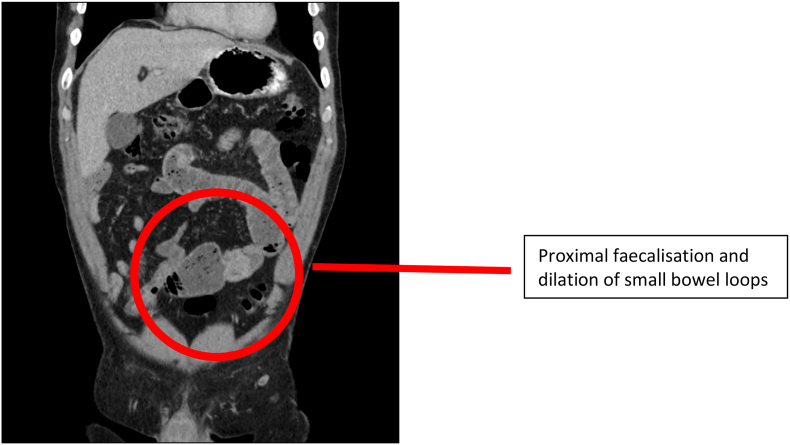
Coronal CT scan of intussusception.
